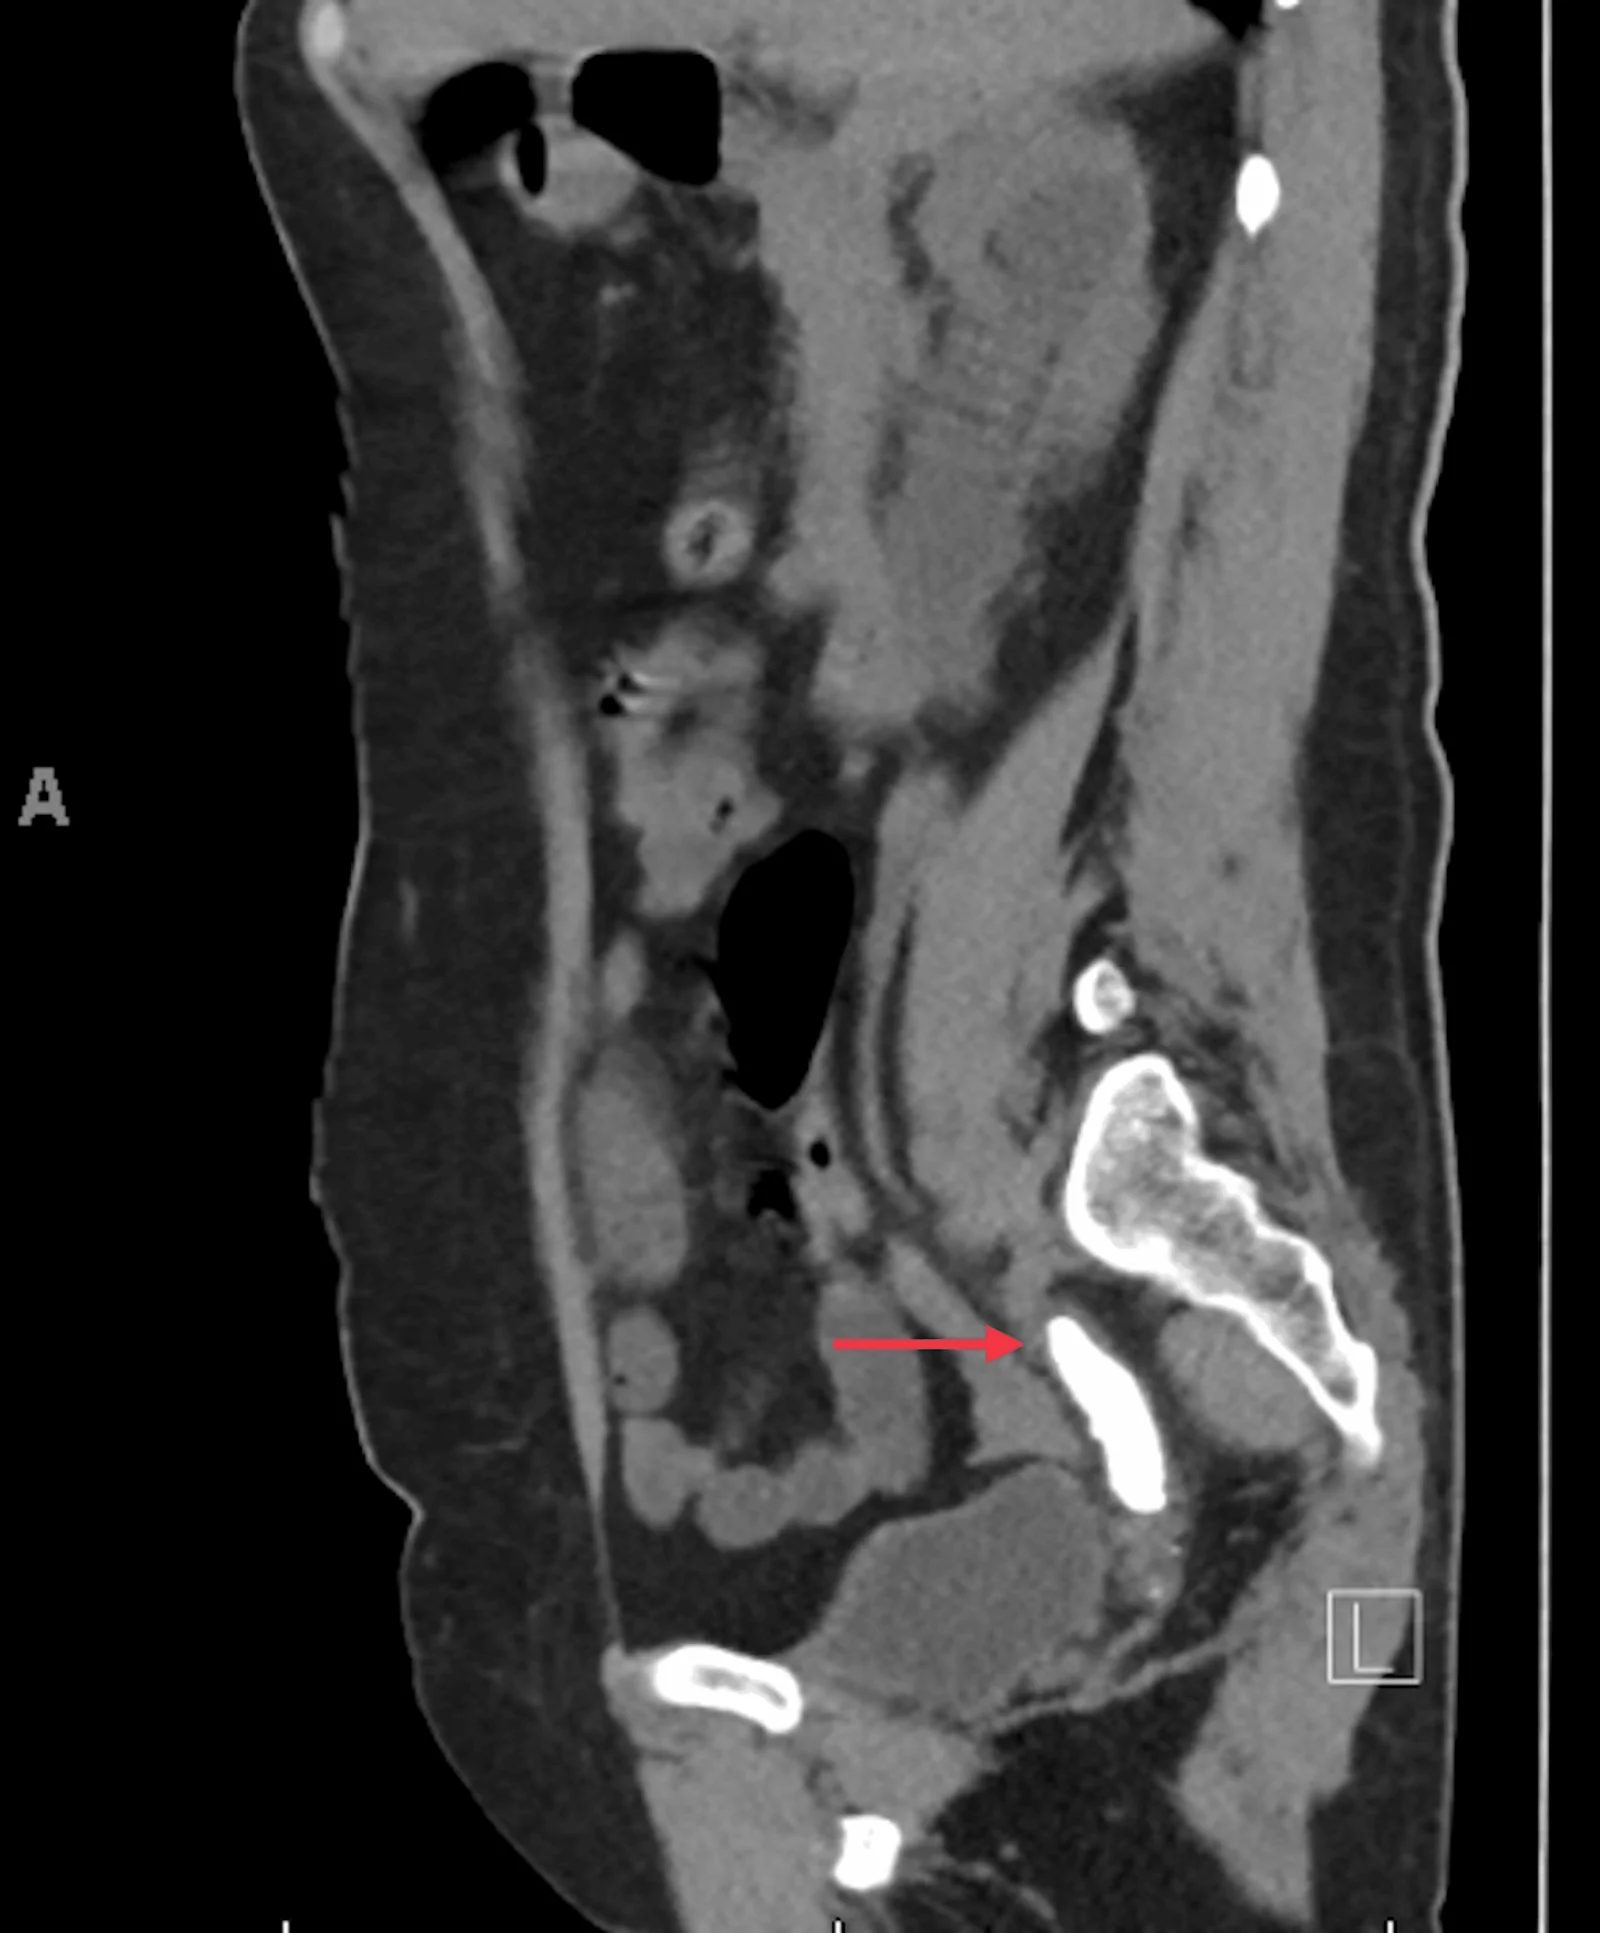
Sagittal non-contrast CT image showing right distal ureteral stones. The red arrow points to the stone.
